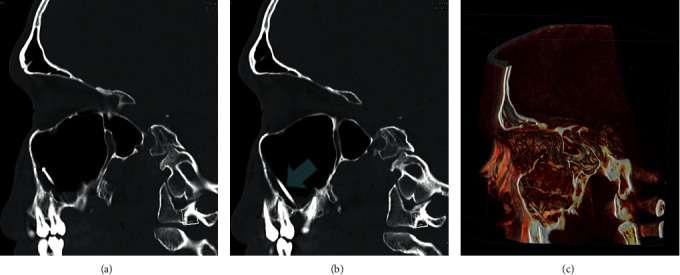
Sagittal cuts of CT sinus showing the head of the foreign body pointing anteriorly, most likely due to the change in position during the MRI. 3D reconstructed CT scan paranasal sinus showing the foreign body.
